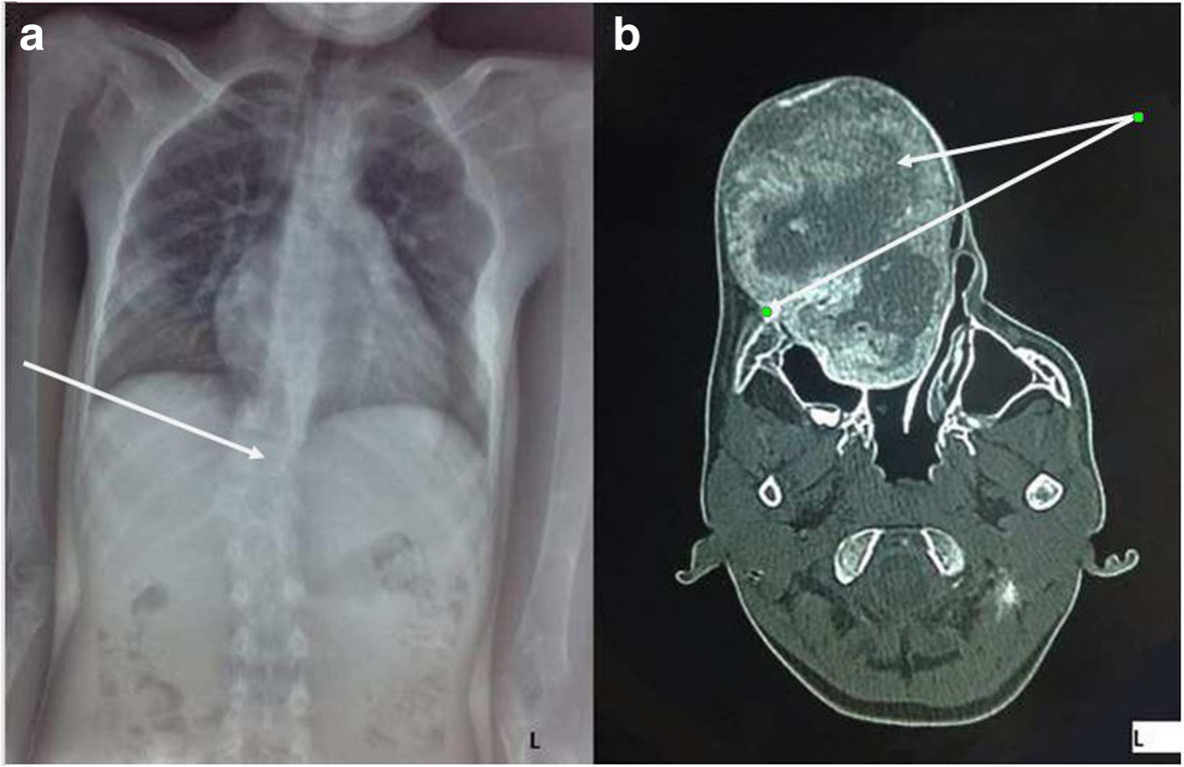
a Radiograph of the chest (PA view) of case 1 showing bending of the spine to the right (scoliosis). (see arrows) b: A CT-scan (axial cut) of case 1 displaying a mixed density lesion with areas of ground glass appearance (which was deep peripherally and light centrally) that involves the maxilla, palate and zygoma. The anteromedial wall of the right maxillary sinus is compressed laterally reducing the size of the maxillary sinus. The vomer is displaced laterally to the left, and the right nostril is completely obliterated
